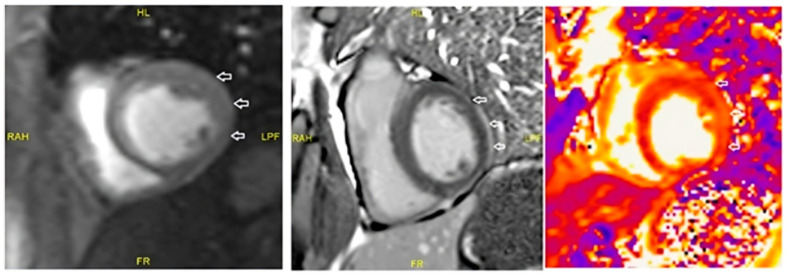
Case 3. Cardiac MRI revealed subepicardial linear contrast enhancement in the lateral wall of the left bentricle (indiacated by an arrow). When evaluated with T2 mapping images, the findings were predominately consistent with a diagnosis of myocarditis, particularly considering the clinical findings. Moderate aortic regurgitation was observed. Secondary casuses, including viral and autoimmune markers, were ruled out.
